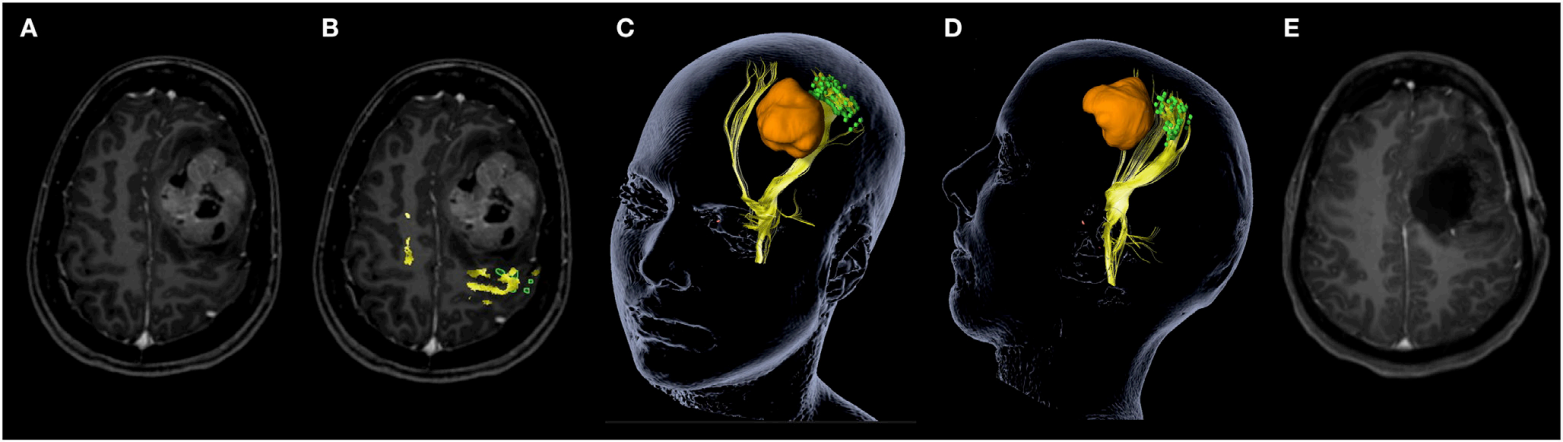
Navigated transcranial magnetic stimulation (nTMS) data clarifies functional anatomy for patient counseling. This screenshot demonstrates how nTMS data can clarify functional anatomy in this young patient with a large Ewing sarcoma metastasis. Tumor location is shown on contrast-enhanced magnetic resonance imaging (MRI) without (A) and with nTMS-positive motor areas (green) including nTMS-based diffusion tensor imaging fiber tracking of the corticospinal tract (yellow) (B). The 3D reconstruction (C,D) shows the same coloring including visualization of the tumor in orange, which allows optimal patient counseling explaining functional anatomy in relation to the tumor. Postoperative MRI scan shows gross total resection (E).
